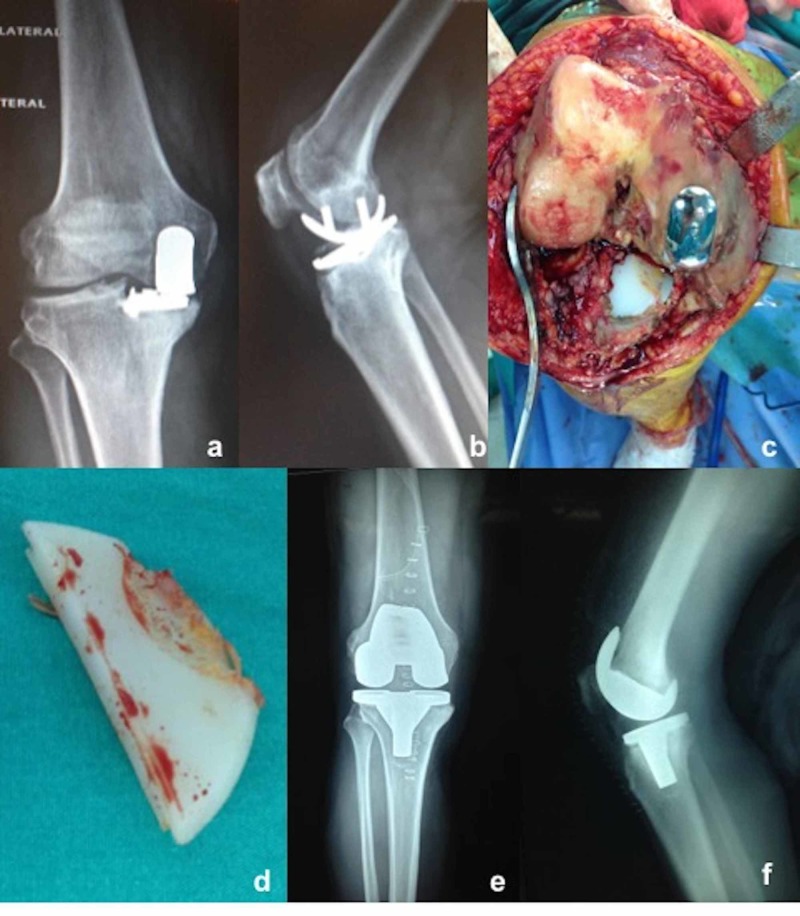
a,b) Tibial and femoral component mismatch (lateralized femoral component and medialized and small tibial component); c) Intraoperative view of the joint; d) Excessive wear on the medial aspect of bearing; e,f) Radiographs after conversion to TKA TKA: total knee arthroplasty
